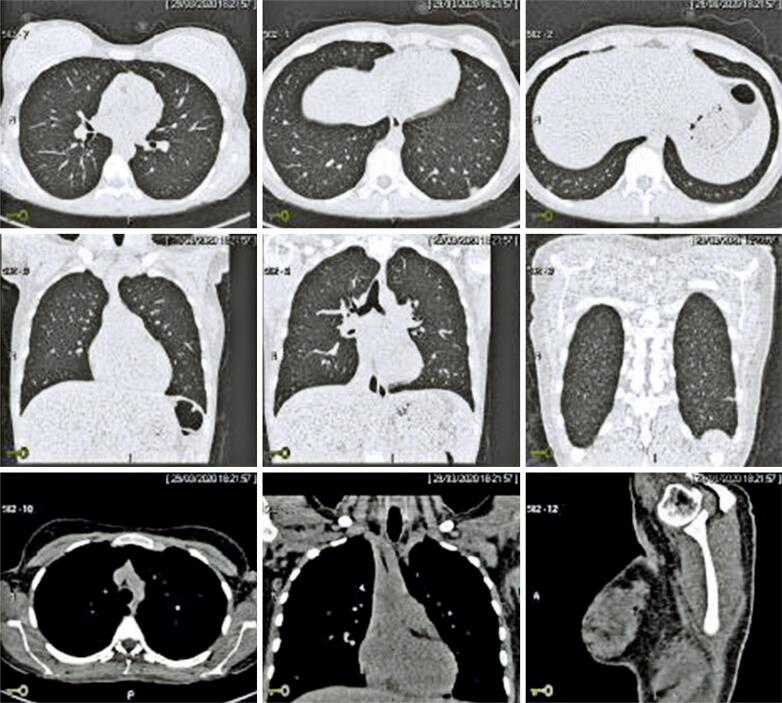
Multislice helical high-resolution computed tomography with no use of intravenous contrast. Chest and lungs images suggestive of pulmonary infection
